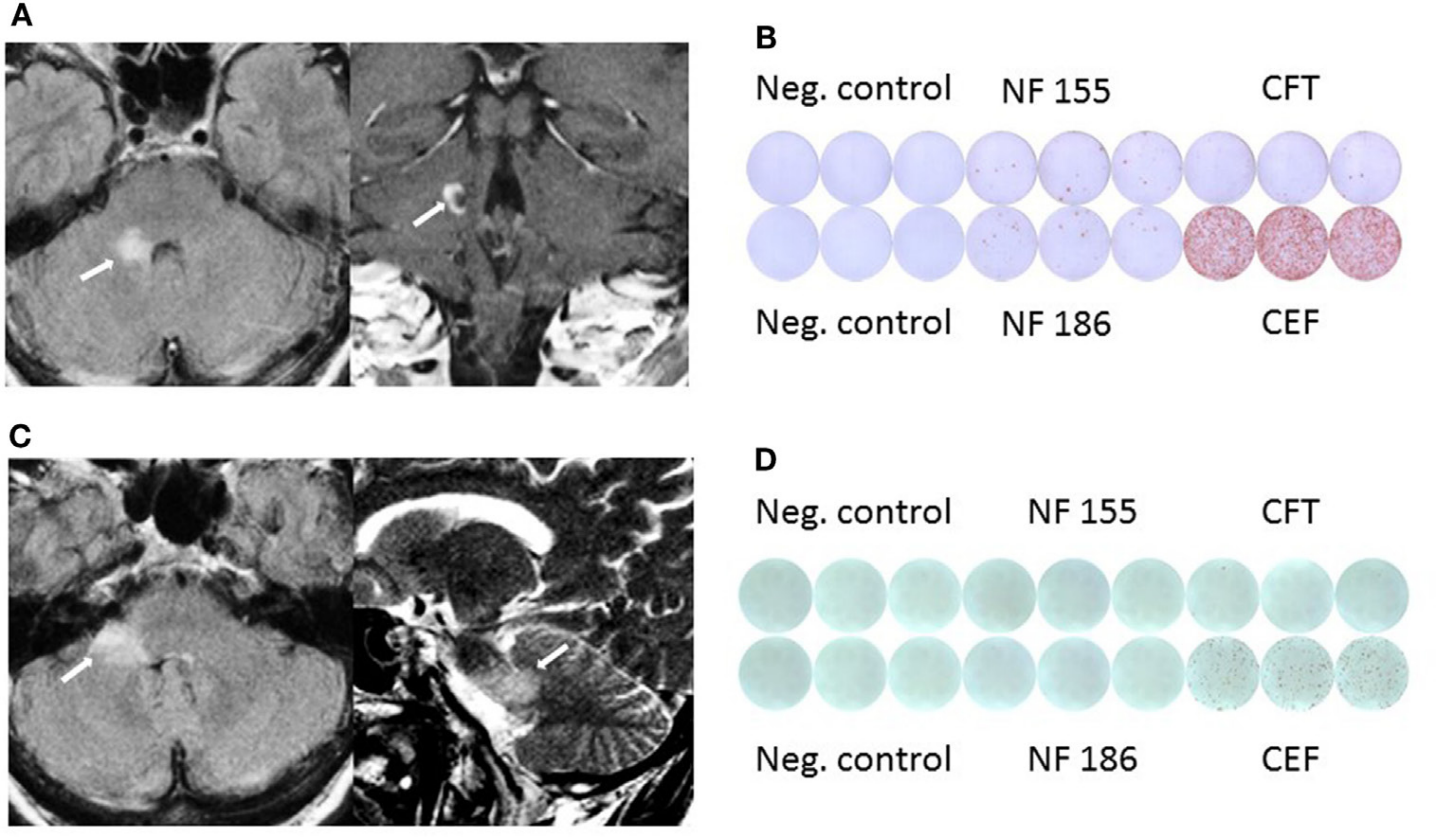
(A) MRI shows pontocerebellar lesion with incomplete peripheral enhancement. (B) Elevated IFNγ response against neurofascin (NF)155 and NF186 before clinical manifestation in Elispot assay. (C) Follow-up MRI shows new lesion in the middle cerebellar peduncle and adjacent pons without clinical manifestation or contrast enhancement. (D) Follow-up Elispot assay shows no IFNγ response against NF.
